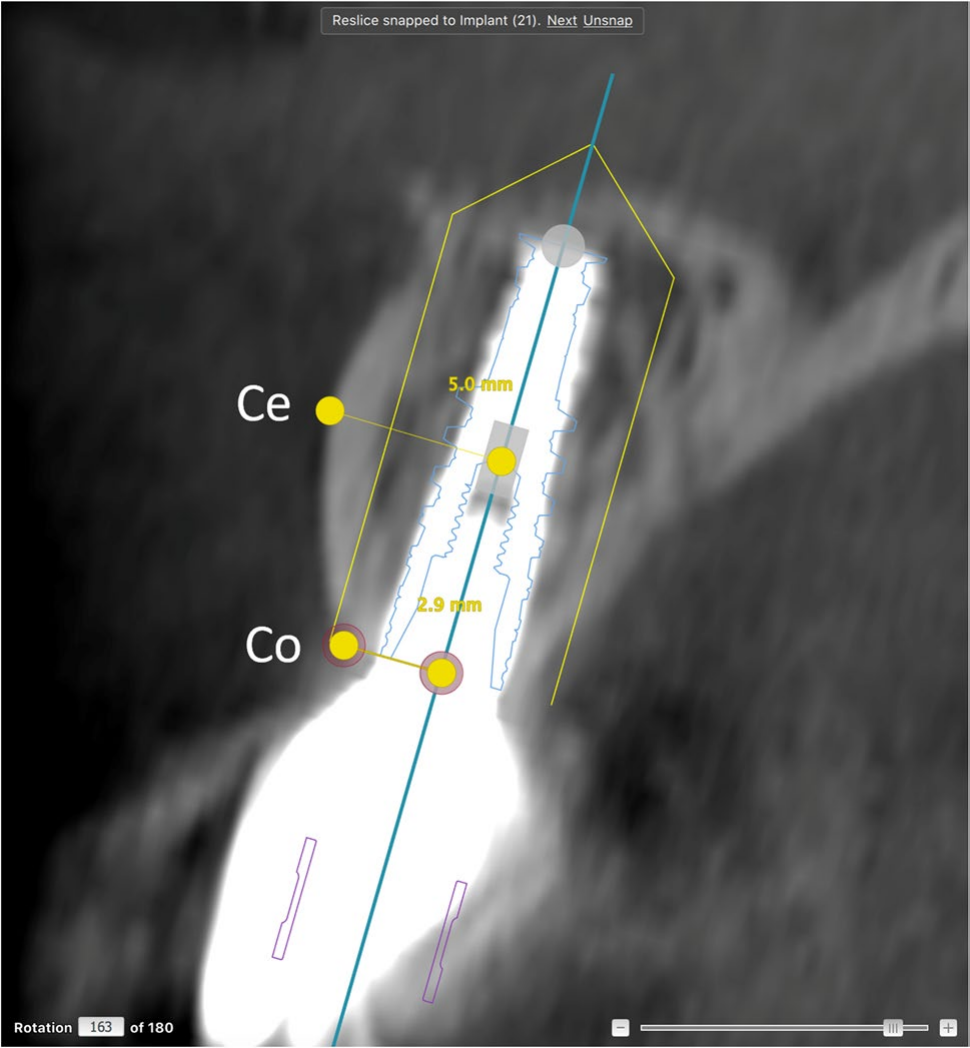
Follow-up CBCT examination in a reconstructed patient. The NobelClinician® software planning instrument was superimposed on the actual placement of the implant. Measures of buccal bone were performed at the central (Ce) and coronal (Co) levels of the implant, taking differences between the actual and the planned implant placement into consideration. In this case, the planned and the actual placement corresponded to one another
